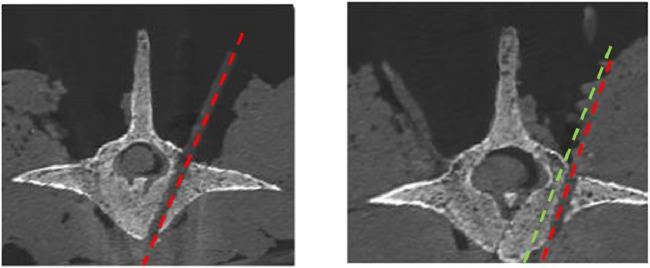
Examples of the drilled screw path on postoperative CT with a case of Grade A and Grade B.
